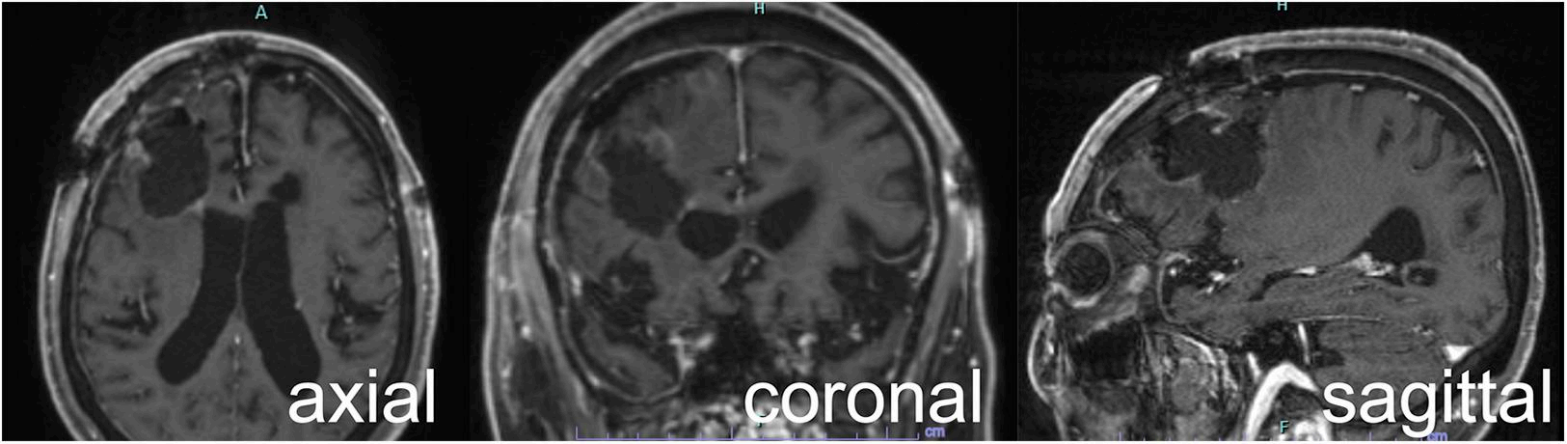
Postoperative contrast-enhanced MRI showing near-total resection of the enhancing lesion. MRI, magnetic resonance imaging.
